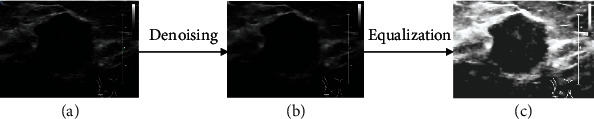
The result after using SRAD filter and histogram to denoise and equalize the breast ultrasound images: (a) shows the original image, (b) shows the denoised image, and (c) shows the result after equalization.
